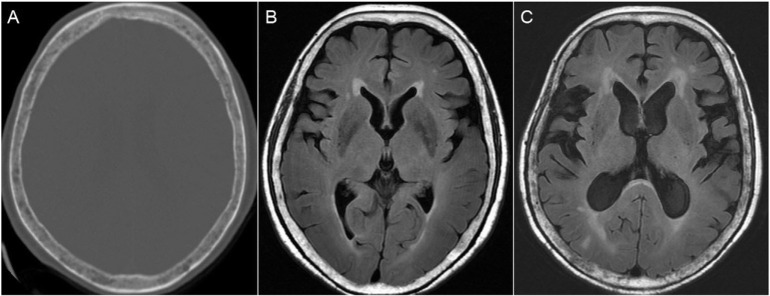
(A) Axial brain CT images showing osteolytic lesions in the skull. Brain MRI of the patient at presentation (B) and four months later (C) showing progressive hydrocephalus and prominent skull bone lytic lesions.
